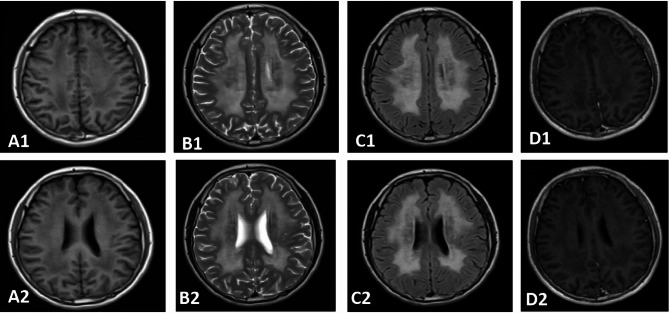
Brain MRI findings. A1,A2:T1-weighted head MRI; B1,B2:T2-weighted head MRI; C1,C2:T2flair head MRI; D1,D2: Enhanced MRI
